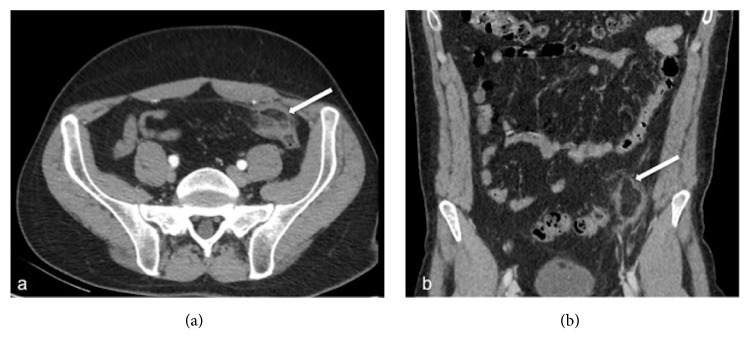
Axial (a) and coronal (b) contrast enhancement CT images show an oval pericolonic fat-density nodule (arrow) with a hyperdense ring and surrounding inflammation.
